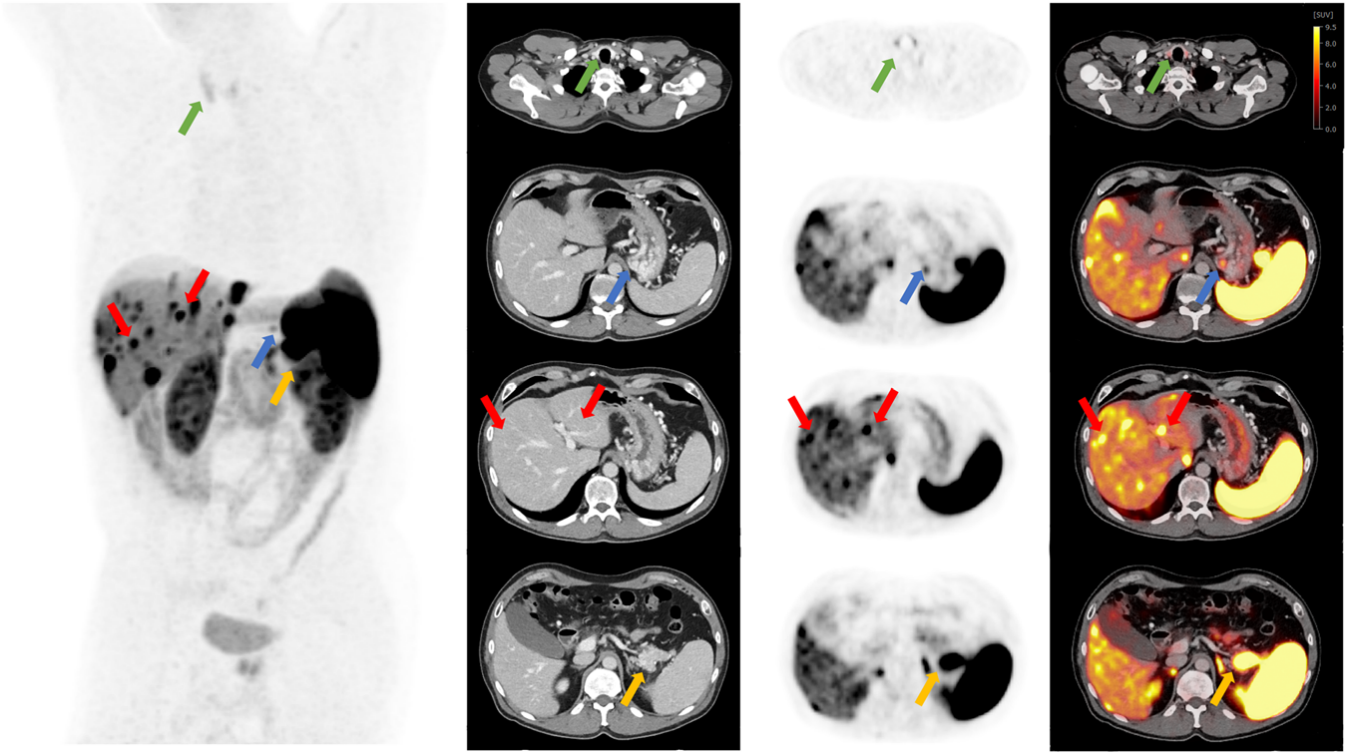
Detailed PET/CT images from a 55-year-old man with a known neuroendocrine tumor of the pancreas (G1, Ki-67 1–2%), diagnosed two years ago. He received systemic chemotherapy (Capecitabin/ Temozolomid and FOLFOX) and now presented with stable disease under therapy with Somatuline. The patient received contrast-enhanced diagnostic CT. In the first row, the thyroid gland and in the second to fourth image series, the upper abdomen is depicted. From left to right: MIP (Maximum Intensity Projection), CT component, PET component, fused CT and PET images of [^18^F]SiTATE PET/CT-scan. In the first row, [^18^F]SiTATE PET shows low uptake in the thyroid gland with no suspect finding on the axial CT, classified as SSTR-RADS 1 (green arrow). In the subsequent row, a lesion in the cardia of the stomach with no suspect finding on the axial CT was classified by one experienced reader and one inexperienced reader as SSTR-RADS 3 A (blue arrow) “Suggestive of, but not definitive for, NET—further workup might be required”. The patient received a gastroscopy, which attested to a non-erosive gastroesophageal reflux disease. [^18^F]SiTATE PET showed intensive uptake in the liver, but three readers could not identify any corresponding finding on CT, so this lesion was classified SSTR-RADS 4, except for one reader, who categorized this lesion as SSTR-RADS 5 (red arrow). Intense uptake in the pancreas with corresponding findings on CT can be found in the last row on axial CT, [^18^F]SiTATE PET, and fused PET/CT, so this lesion was classified SSTR-RADS 5 by all four readers (yellow arrow). All readers rated the uptake intensity as sufficient for recommending PRRT according to the SSTR-RADS 1.0 criteria
